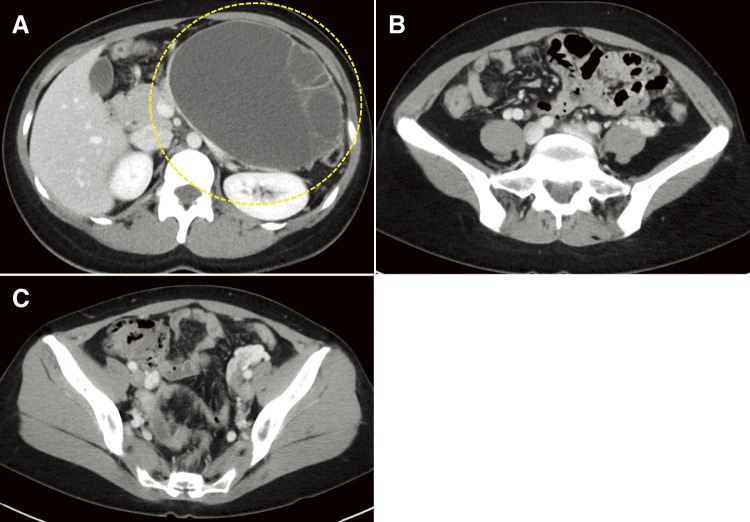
The CECT findings 10 months after the first examination: (A) A multifocal cystic mass measuring 150 mm in length was observed in the pancreatic tail (dotted line), demonstrating a tendency to enlarge mildly. (B, C) No ascites were observed. CECT, contrast-enhanced computed tomography
